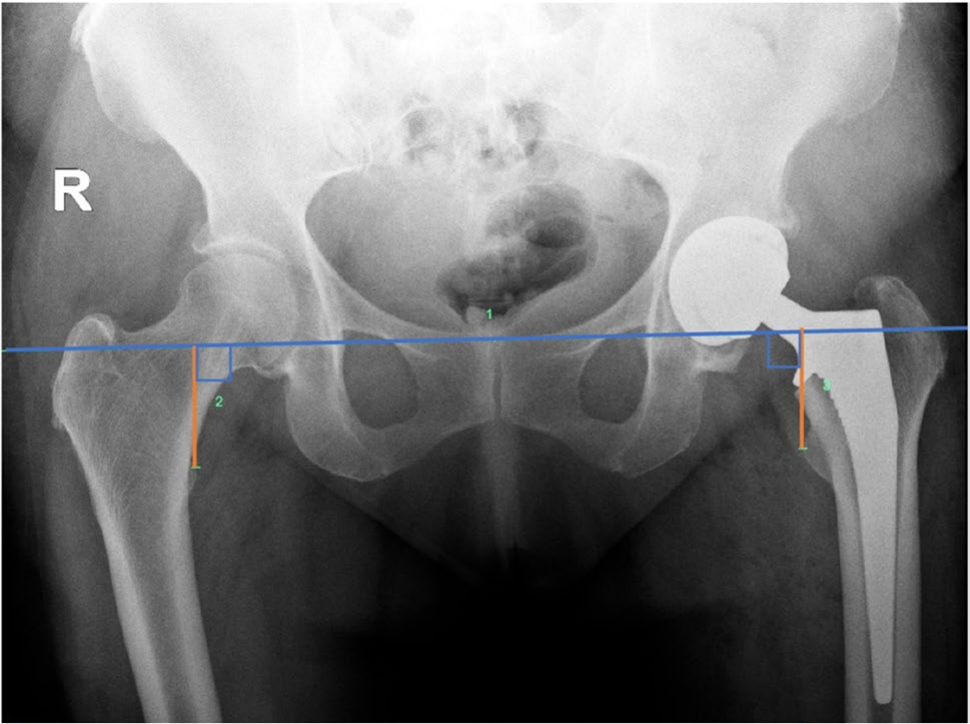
Anteroposterior pelvic radiograph demonstrating measurement of leg length discrepancy according to Woolson’s method which was defined as the perpendicular distance between the inter-teardrop line and the proximo-medial apex of the lesser trochanter (orange line)
